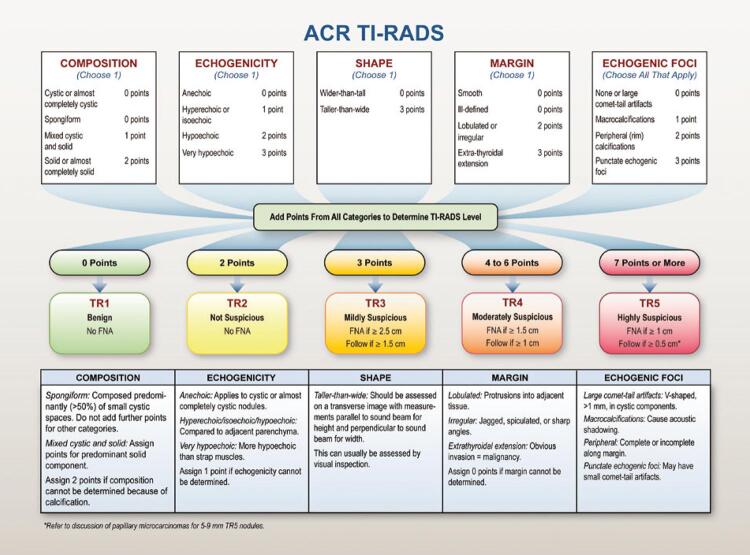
ACR-TR diagnostic matrix for ultrasound classification of thyroid nodules from 2017 White Paper of the ACR TI-RADS Committee
